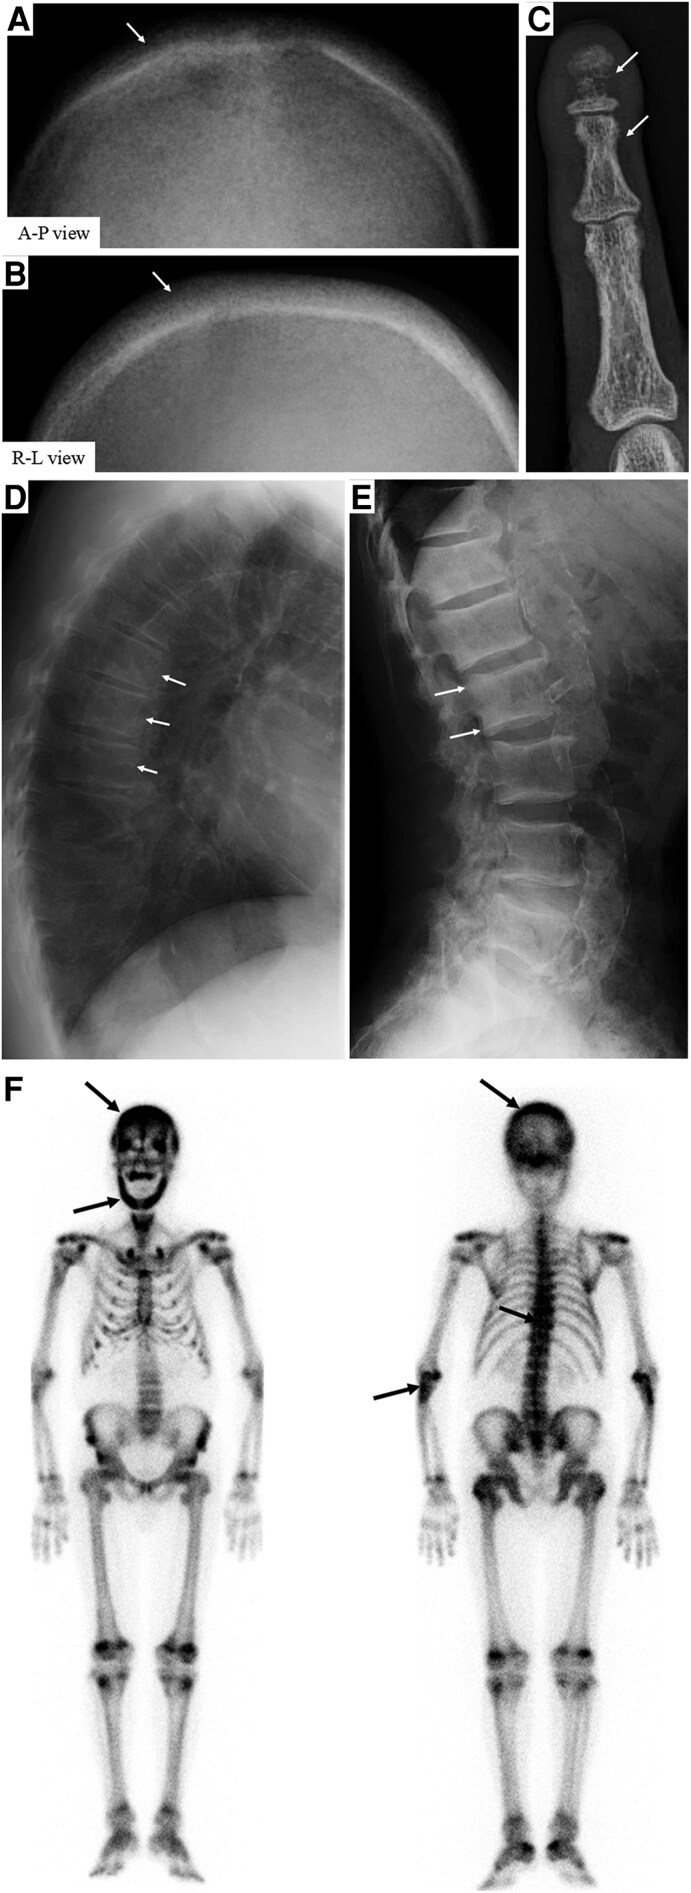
X-ray and bone scintigraphy findings. (A) Anteroposterior view, (B) right-left view. The X-ray examination showed the salt and pepper sign (multiple tiny well-defined lucencies caused by resorption of trabecular bone) in the skull. The margins of the skull were unclear. (C) Bone erosions and bone resorption (arrow) were noted in the second distal and intermediated phalangeal bones of the hand. (D) Kyphosis (arrow) of the thoracic spine was noted. (E) The lumbar spine showed the rugger jersey sign (defined by the sclerotic bands [arrows] on the inferior and superior endplates of the vertebral bodies and the alternating dense [arrow] to lucent-dense [arrow] appearance). (F) Bone scintigraphy showed a high uptake in the skull (arrow), thoracic and lumbar spine (arrows), and many joints (arrows).
